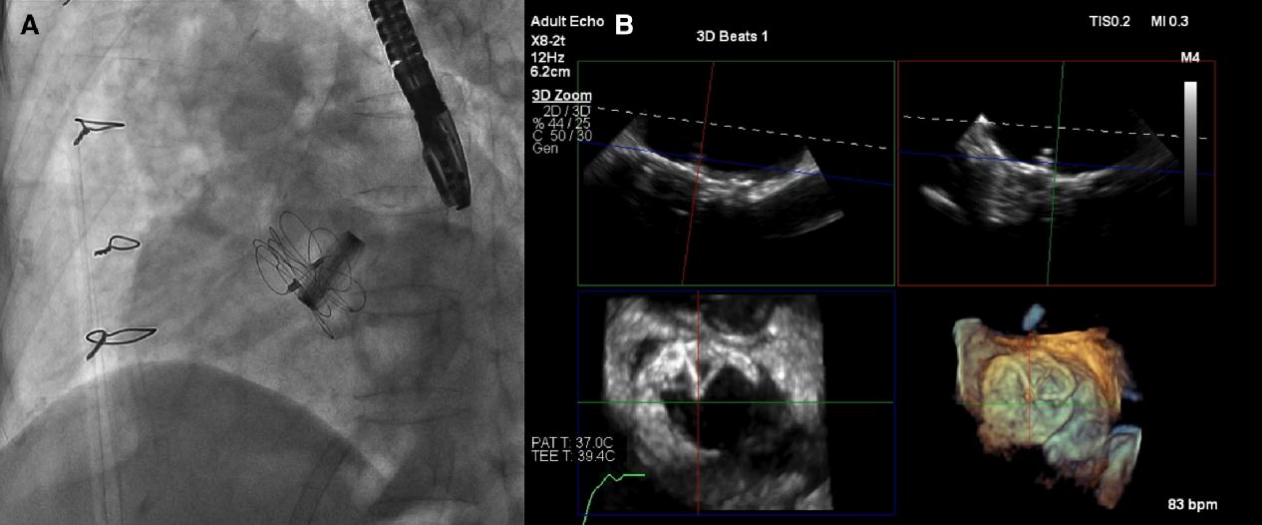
Closure with 37 mm GORE CARDIOFORM ASD Occluder device. Videos of (*A*) and (*B*) are also provided in Supplementary material online, *Video S2A* and *B*. (*A*) Angiogram. (*B*) Transoesophageal echocardiogram.
